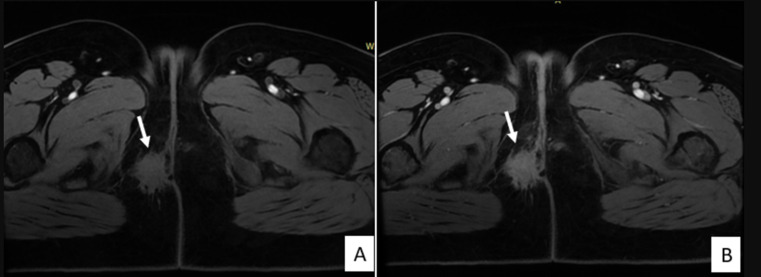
on the T1-weighted fat-suppressed sequence (A); the lesion appears isointense relative to skeletal muscle, containing small hyperintense foci; Axial post-contrast T1 fat-saturated image showing no enhancement after gadolinium injection (B)
